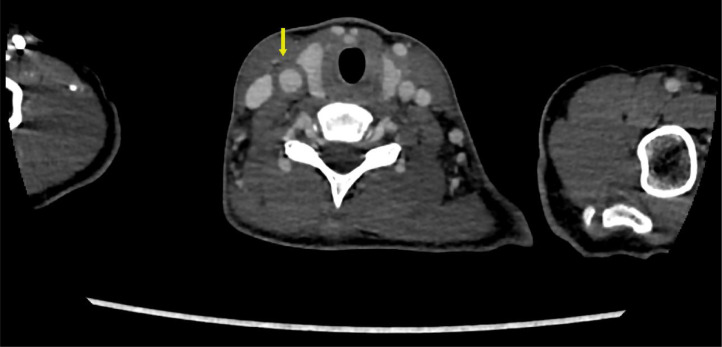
CTA axial view showing a diffusely thickened wall of right CCA (arrow).
